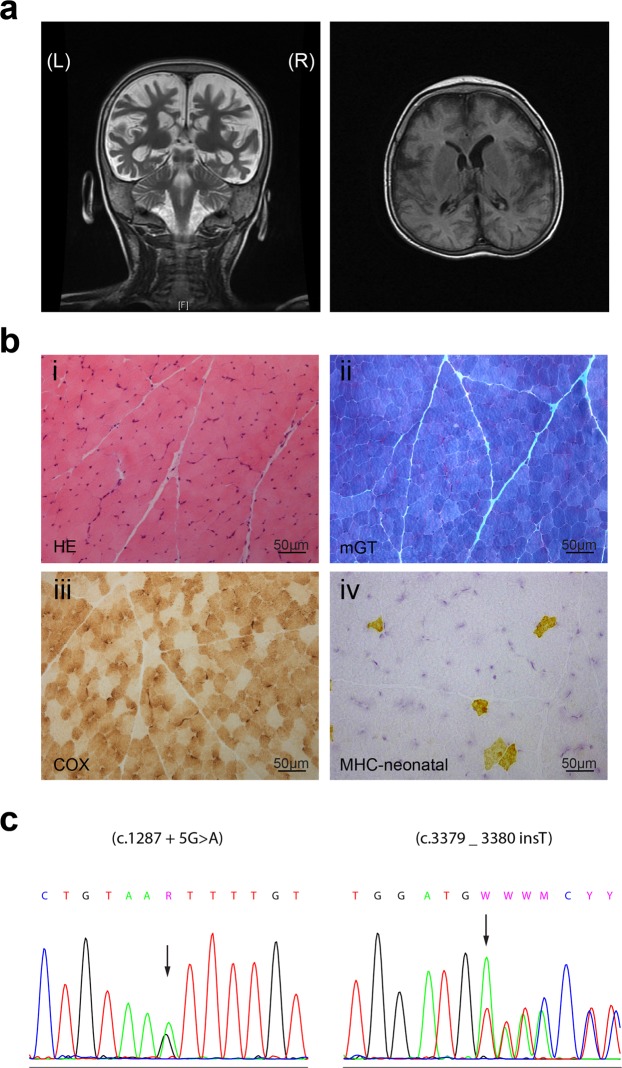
Brain and muscle abnormalities in subject S1 at age 3 and 4 years. (a) Brain magnetic resonance imaging of the individual at the age of 3. Note cortical atrophy and reduced white matter volume in both coronal T2-weighted (left panel) and axial T1-weighted (right panel) images. (b) Muscle biopsy was performed at the age of 4 years and showed minimal myopathic changes consisting of mild variation in muscle fibre diameter (i, ii), a population of fibres with weak cytochrome c activity (iii) and a few fibres of normal size positive for neonatal myosin (iv).
